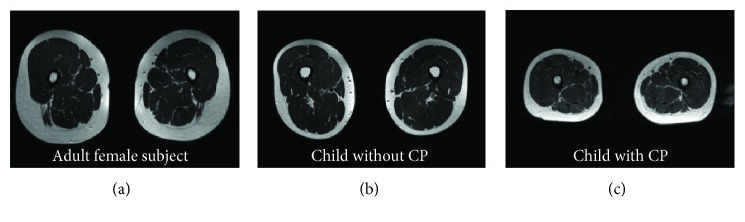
Representative MR image of the shank of (a) adult female subject, (b) child without CP, and (c) child with CP.
